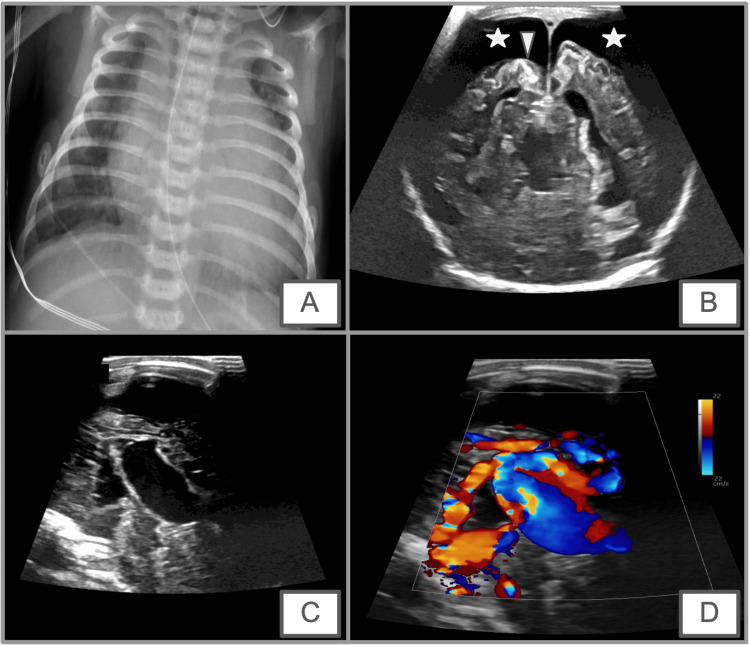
A frontal chest radiograph, brain ultrasound, midline sagittal grayscale, and color Doppler images. (A) A frontal chest radiograph obtained on DOL #11 shows a significant enlargement of the cardiac silhouette and mild enlargement of central pulmonary vasculature, which may be related to extra-cardiac shunting. (B) Coronal grayscale image from brain ultrasound obtained on DOL# 14 shows a significant parenchymal volume loss with enlargement of the extra-axial space (star). (C and D) Increased echogenicity of the frontal cortex (arrowhead) suggests laminar necrosis in the setting of prior infarction. Midline sagittal grayscale (C) and color Doppler (D). DOL: day of life
